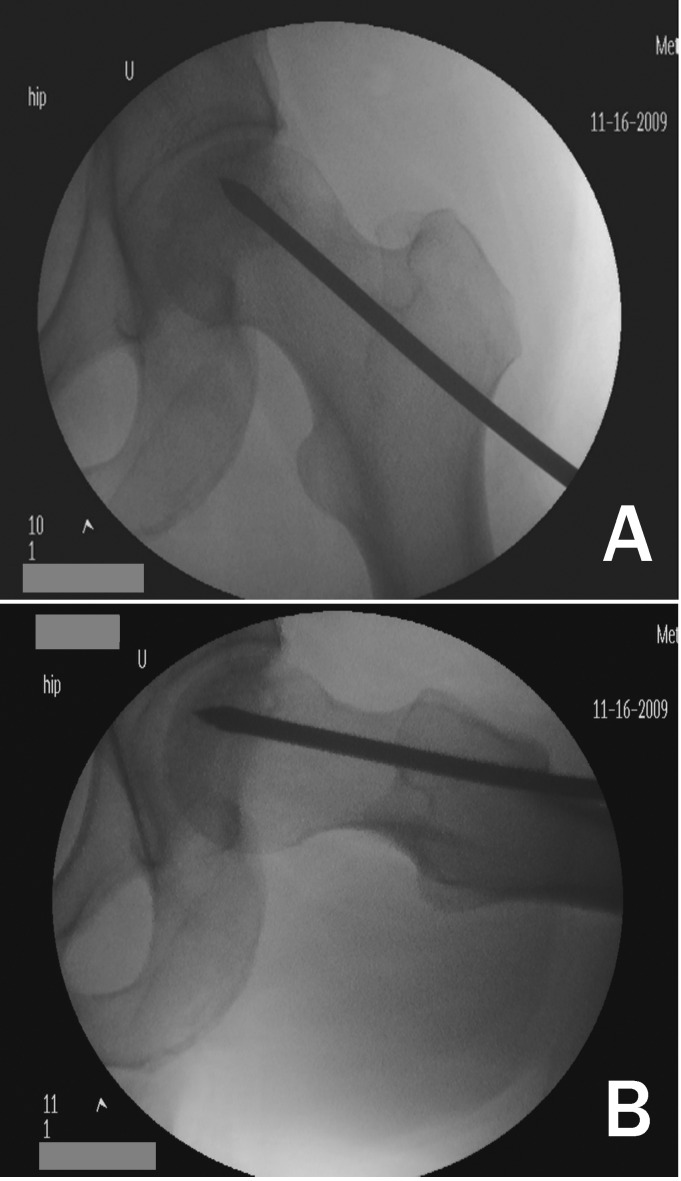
Anteroposterior (A) and frog-leg lateral (B) radiograph showing the decompression trochar in the area of necrosis (published with permission from Sierra RJ). Alternative procedure for osteonecrosis of the femoral head, Figures 3A and 3B (24).
